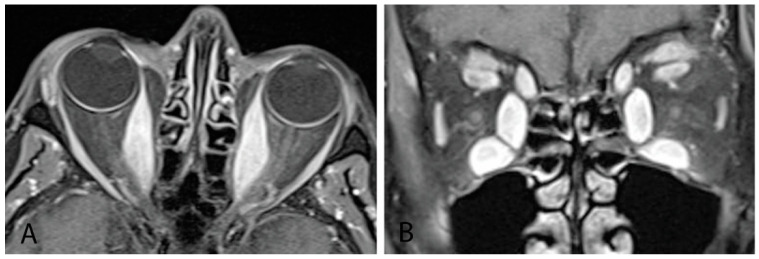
(A) MRI orbits with gadolinium with sagittal cut showing thickening of bilateral medial recti muscles. (B) Coronal cut from same patient showing thickening of all bilateral inferior, medial, and superior recti muscles, as well as levator palpebrae superioris and superior oblique muscles.
